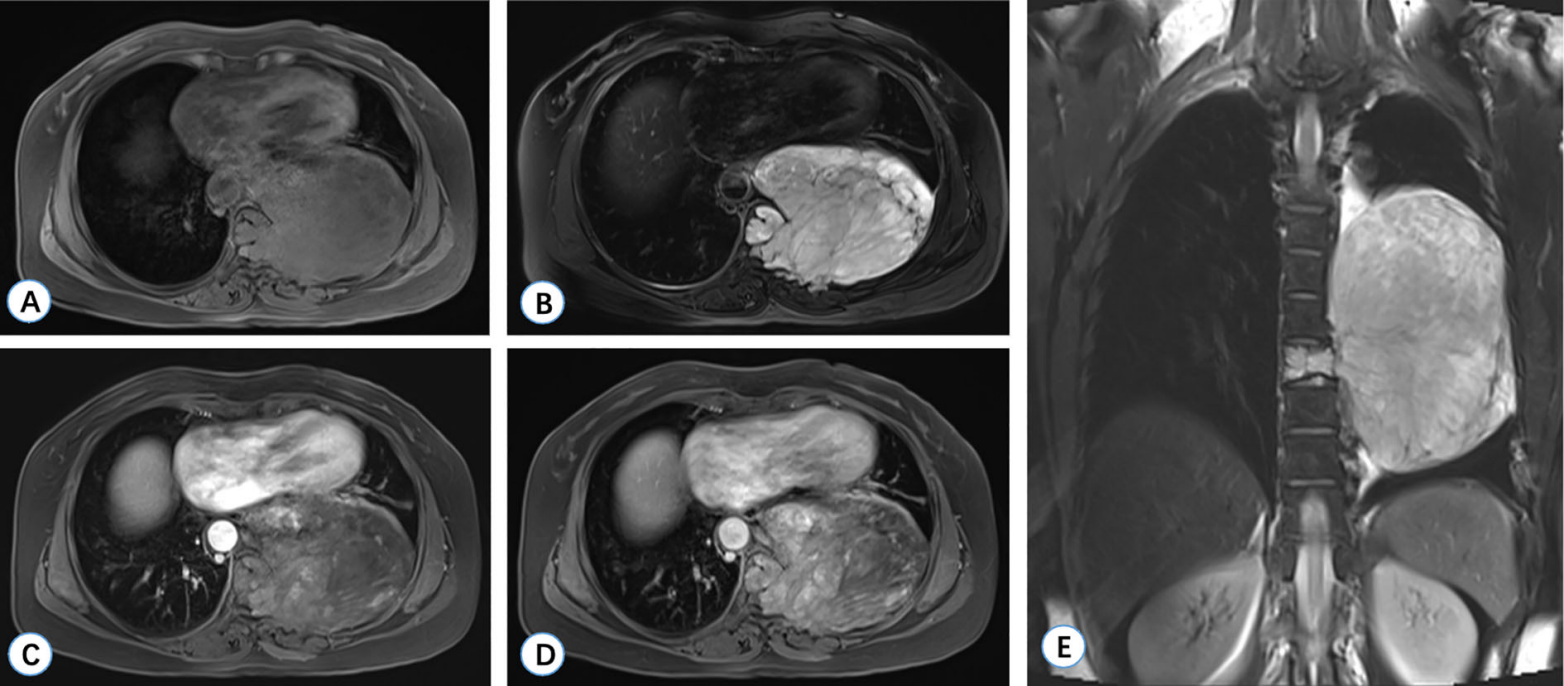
MRI manifestations of Case 2 (T8 vertebra). (A) An axial T1-weighted image reveals a large lobulated hypointense mass at the T8 vertebra, protruding into the thoracic cavity on the left side. (B) An axial T2-weighted image demonstrates heterogeneous hyperintensity of the tumor. (C, D) Enhanced T1-weighted images indicate mild irregular enhancement. (E) A coronal T2-weighted image shows that the tumor originates from the T8 vertebral body.
